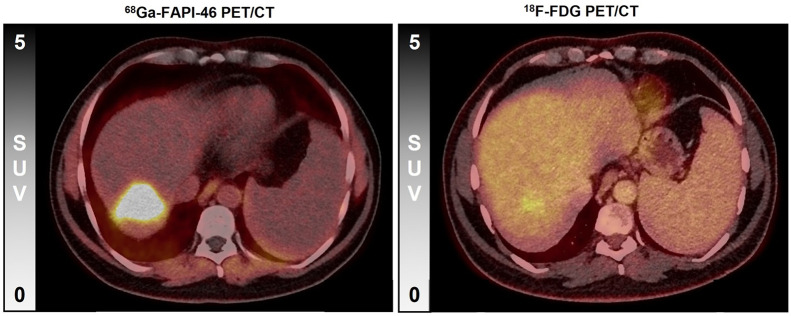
Intrahepatic primary tumor of patient 4, demonstrating 18.7-fold higher tumor-to-background uptake ratio in ^68^Ga-FAPI-46 PET (31.8) than in ^18^F-FDG PET (1.7).
